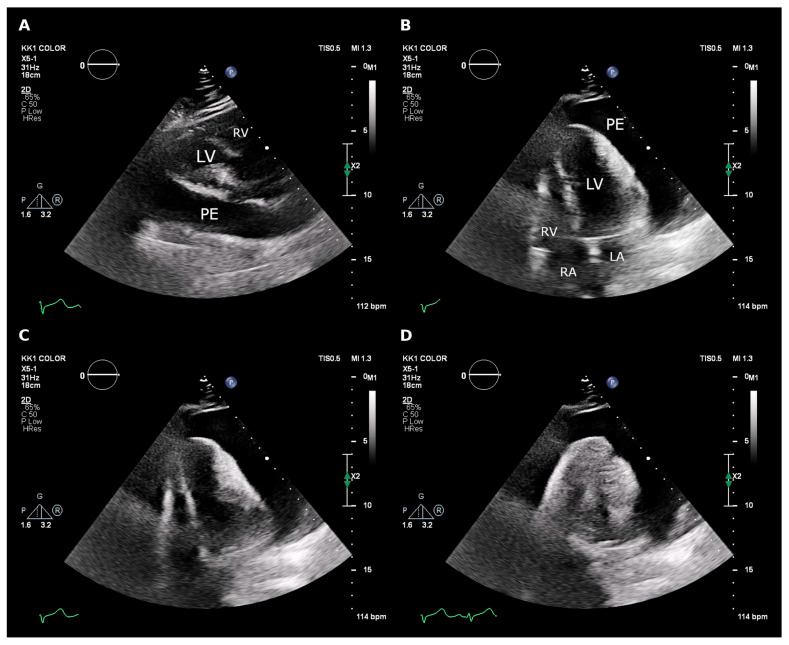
Preoperative transthoracic echocardiographic features of cardiac tamponade in a 29-year-old woman at 30 + 4 weeks of gestation (all panels obtained at presentation, before surgical drainage). (A) Parasternal long-axis view demonstrating a large pericardial effusion (PE) measuring up to 30 mm posterior to the left ventricle. (B–D) Sequential apical four-chamber frames showing a circumferential pericardial effusion of up to 40 mm around the apex and lateral wall, with diastolic collapse of the right atrium and right ventricle. LA, left atrium; LV, left ventricle; PE, pericardial effusion; RA, right atrium; RV, right ventricle.
